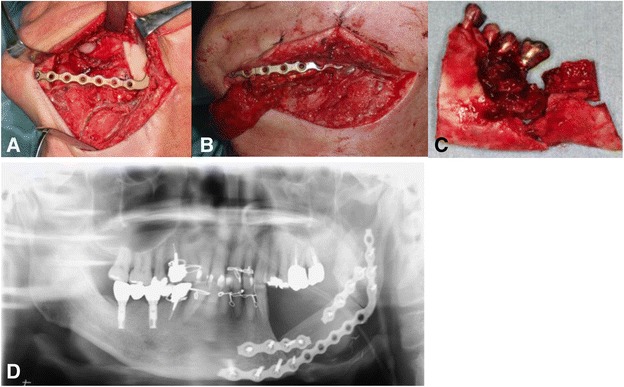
a Intraoperative photo. The affected left mandible was segmentally resected. b Intraoperative photo. A vascularized fibula bone graft. c Resected mandible. d Panoramic X-ray image after the surgery
